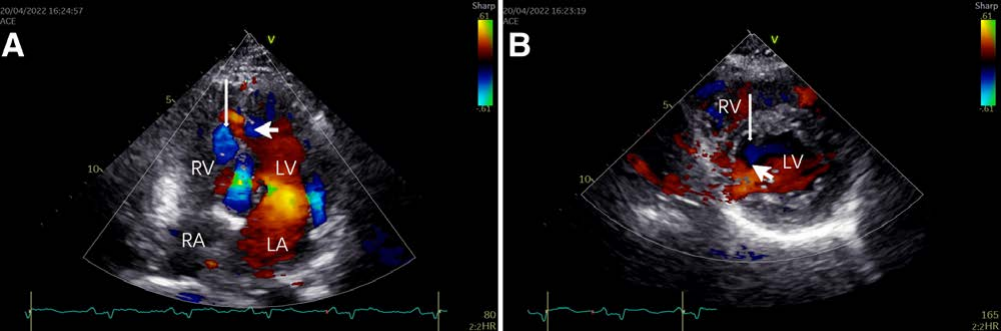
Color doppler flow imaging (CDFI). The apical 4-chamber view, (A) and parasternal papillary muscle short-axis view, (B) with CDFI revealed low velocity bidirectional flow at the continuity interruption (short arrows). The bidirectional flow communicated the bilayer structure of the ventricular septum (long arrows) with the left ventricular cavity.
